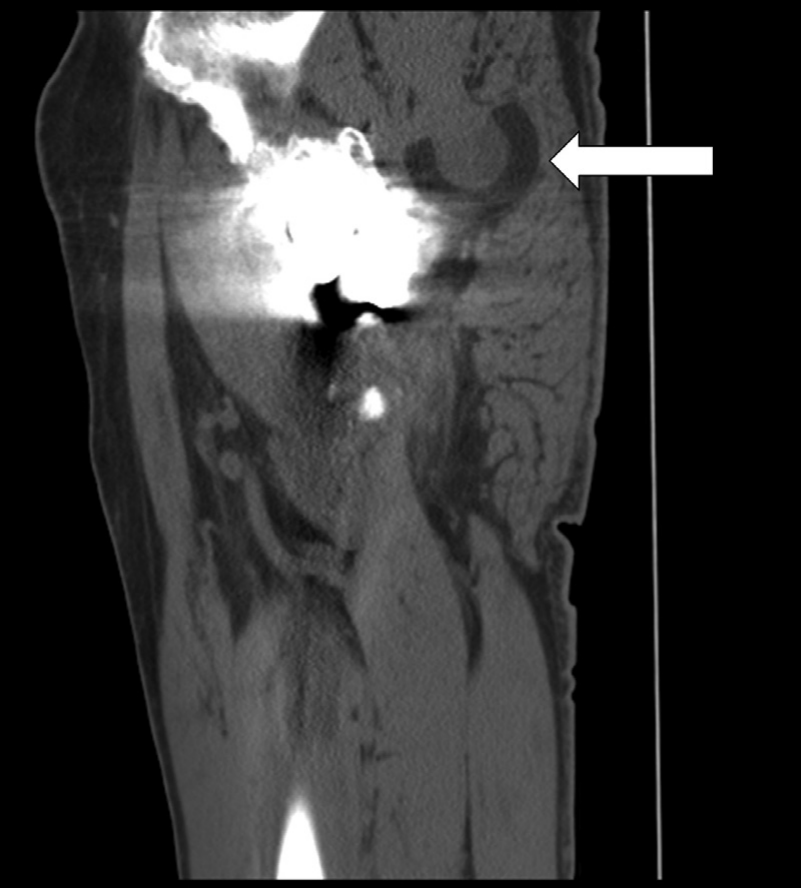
Sagittal CT image confirms that the polyethylene femoral head (arrow) is dislocated posterosuperior in the posterior gluteal musculature. The polyethylene head is inverted. The polyethylene appears intact without focal thinning or fragmentation.
